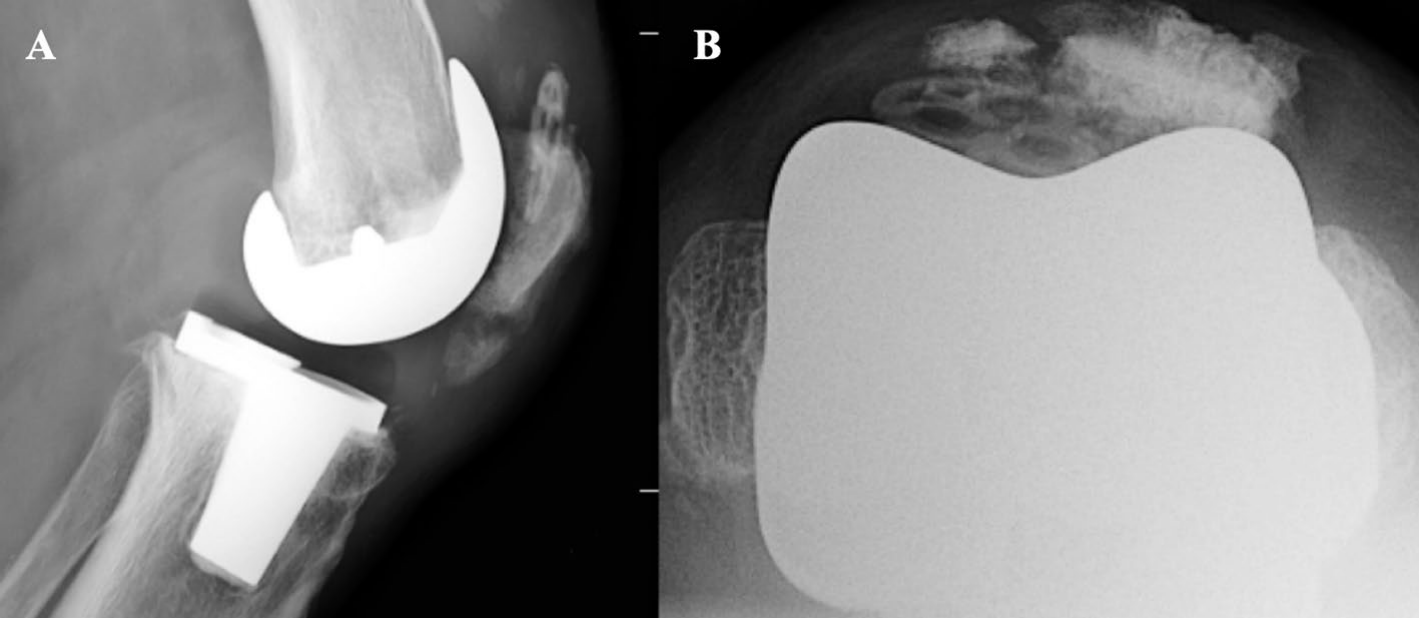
Periprosthetic patellar fracture in the resurfaced setting with loosening of the patellar button but an intact extensor apparatus. A Lateral X-ray view; B Merchant X-ray view
